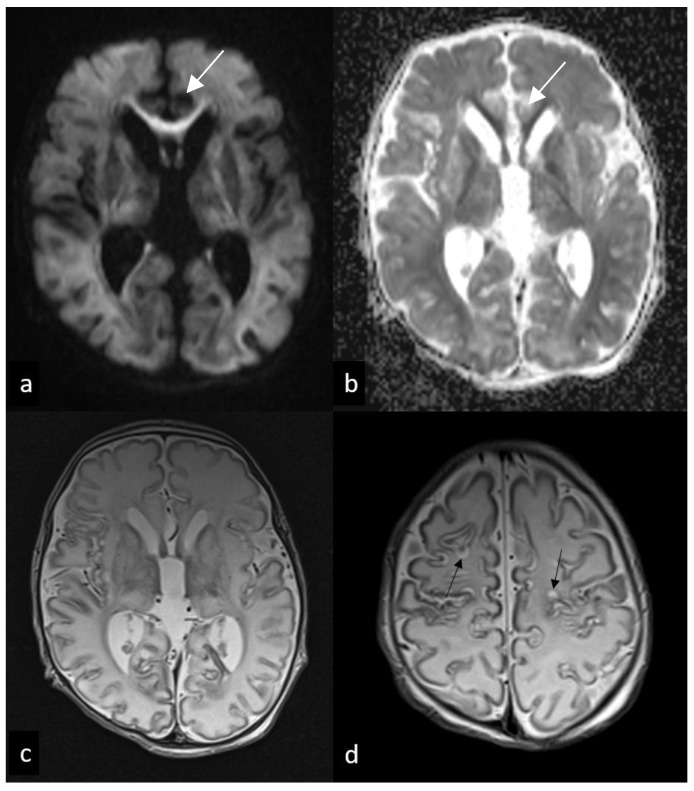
Follow-up MRI performed 7 and 12 days after birth. Axial DWI (a), ADC, (b) and T2WI (c,d) show mild diffusion restriction involving subcortical white matter and corpus callosum ((a,b), arrows), suggesting an acute wallerian degeneration, as well as diffuse ventricular enlargement (c) and subcortical cavitations (black arrows in (d)).
